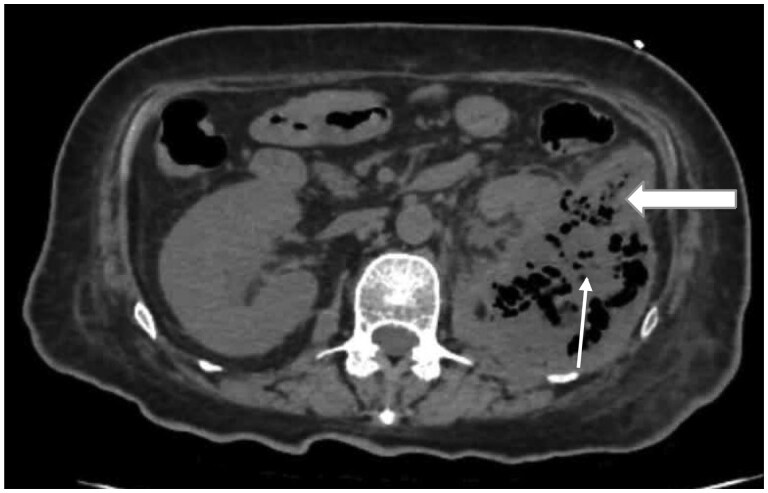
Axial non-contrast CT image demonstrating perinephric air-containing collection (thin arrow) abutting the descending colon with focal mural irregularity (Block arrow) raising suspicion of fistulous communication.
